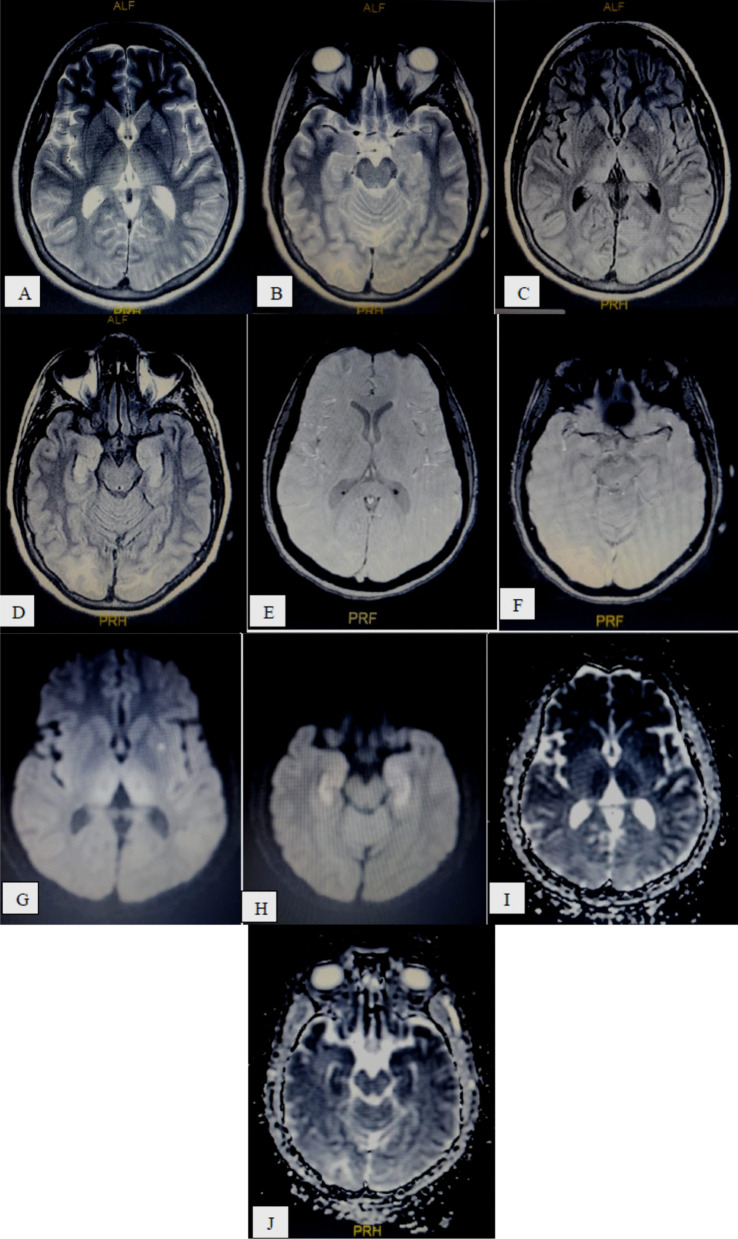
51-year-old-female COVID-19 positive patient presented with impaired level of consciousness, A, B T2 WI, C, D FLAIR, E, F SWI, G, H DWI and I, J ADC map showing bilateral thalamic, bilateral occipital subcortical, bilateral temporal cortical and subcortical T2 and FLAIR hyperintense SI with restricted diffusion (hyperintense in DWI and hypointense in ADC map) represented acute necrotizing encephalopathy without hemorrhage, with left putamen acute infarction
